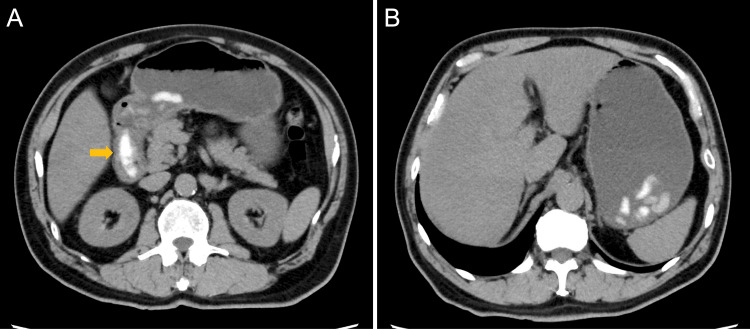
Computed tomography of the abdomen CT of the abdomen showing a high-density content measuring approximately 4 cm in length in the duodenal bulb (A, arrow), along with multiple high-density contents in the stomach and gastric distension (B).
